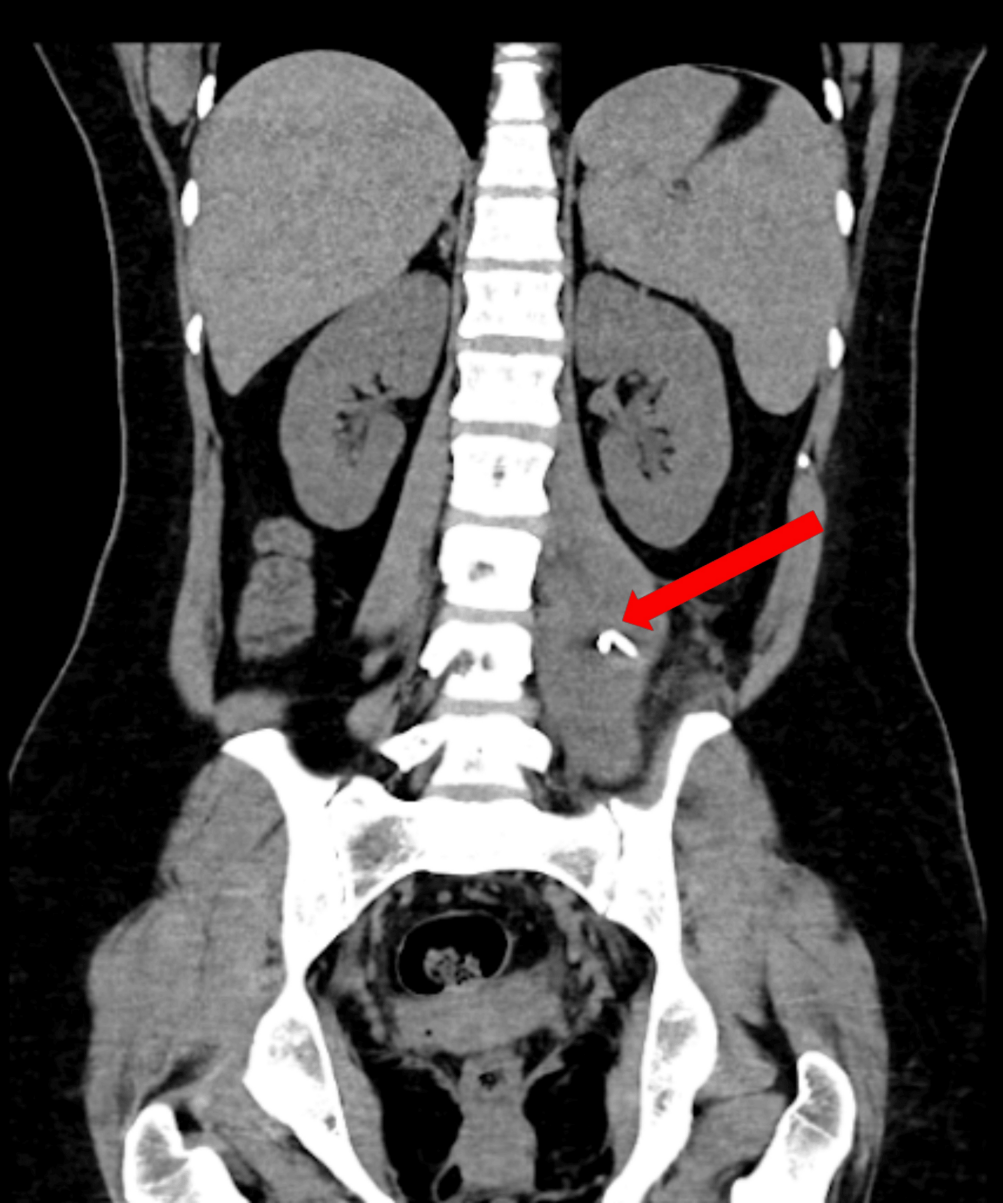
The third CT coronal reconstruction shows a marked reduction in the size of the hematoma, with an estimated volume of 200 cc, and demonstrates the presence of a 12 Fr "pigtail" catheter within it.
